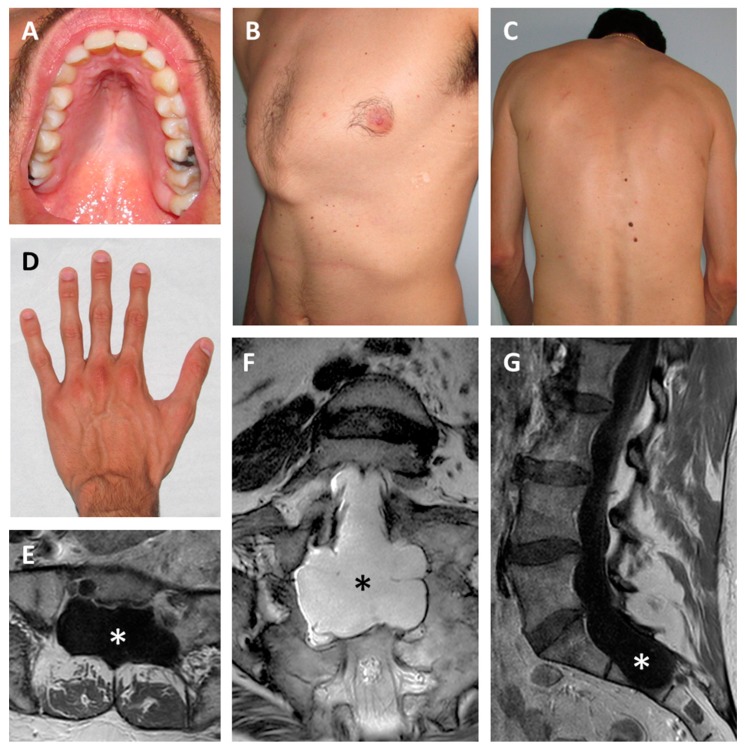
Clinical features. Individual 1 showing high-arched palate (A), pectus carinatum (B), scoliosis (C) and arachnodactyly (D). Lumbar spine MRI of Individual 2 demonstrating bilateral dural ectasias at axial (E), coronal (F), and sagittal (G) views. Asterisks indicate the dilated dural sac.
